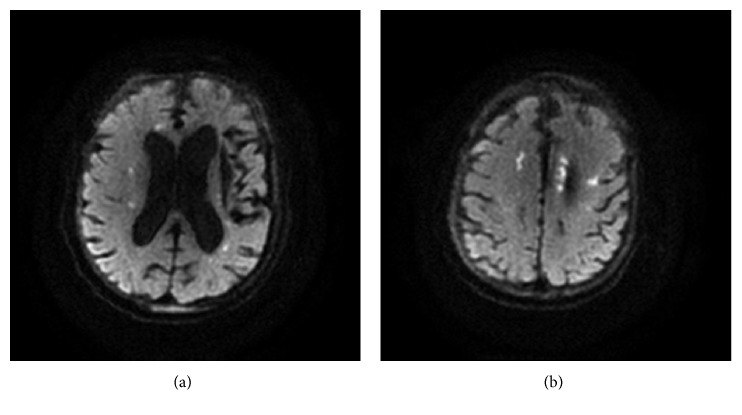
Brain MRI showing small areas of diffusion restriction with T2 hyperintensity over (a) anterior horn of right lateral ventricle and (b) posterior horn of left lateral ventricle and left corona radiata, consistent with acute infarcts.
